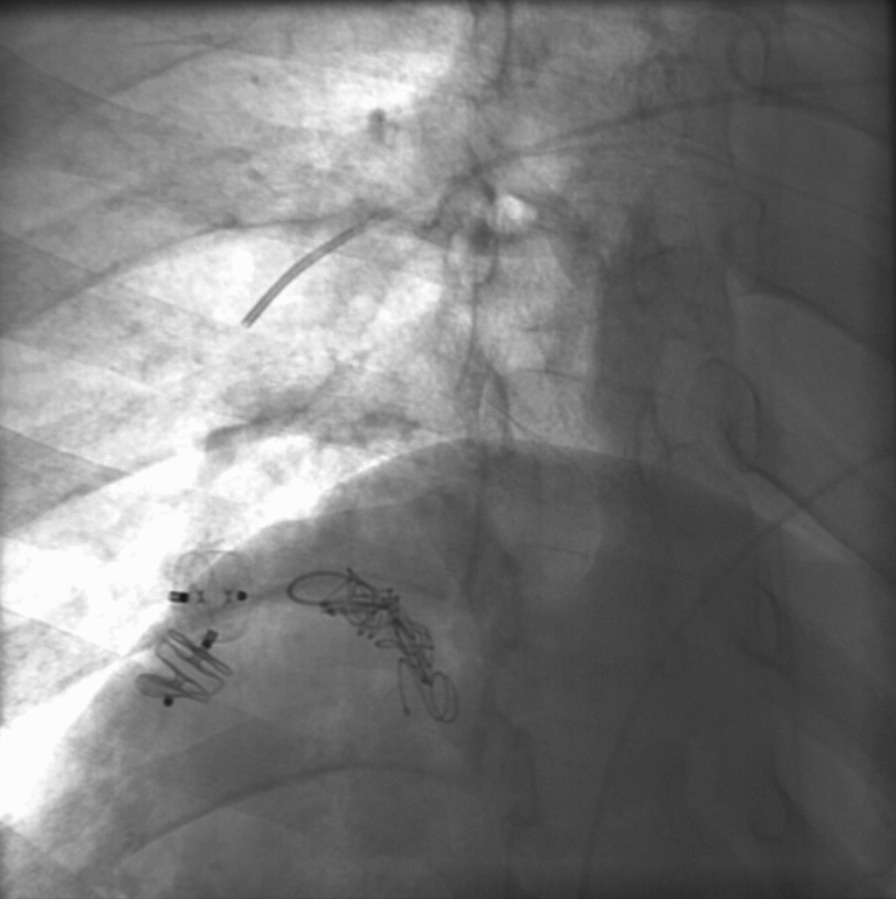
Computed tomography angiogram image showing successful embolization of vasculature feeding the pulmonary arteriovenous malformation using Amplatzer Vascular Plug II
